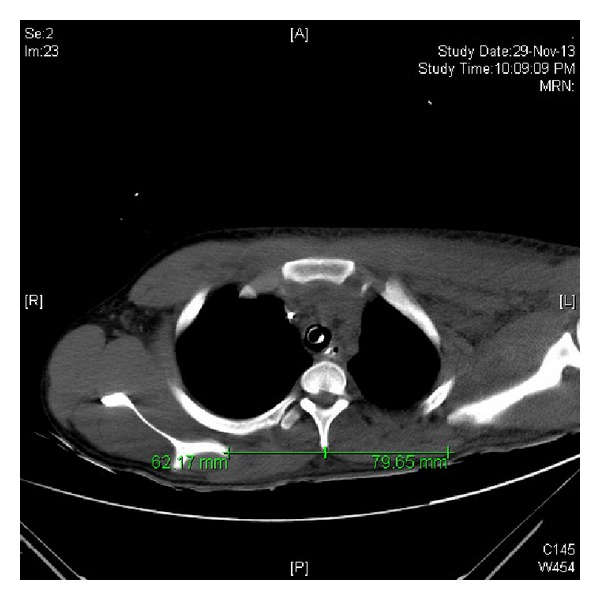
Axial CT section revealing the increase in distance between medial border of left scapula and the spinous process of the fourth thoracic vertebra.
